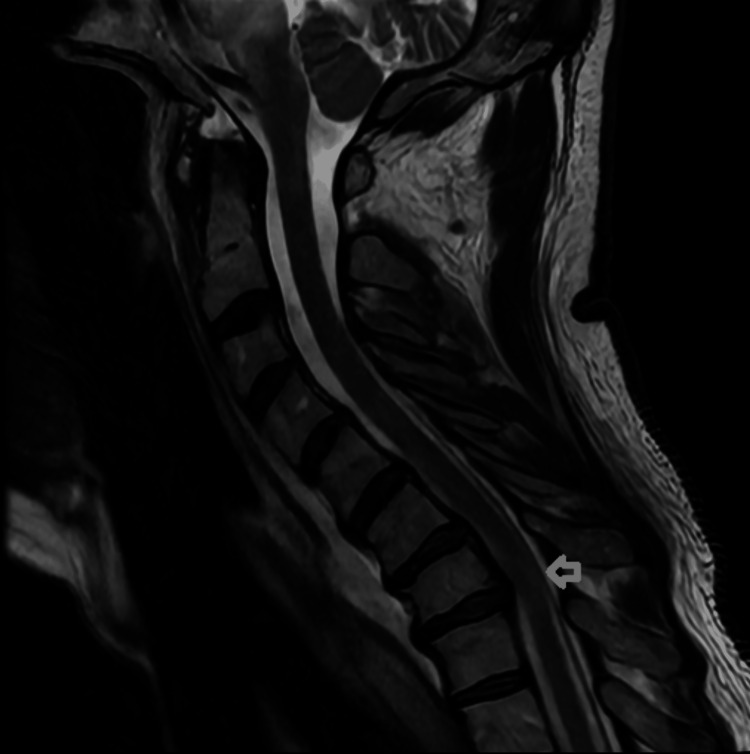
MRI C spine (T2 sequence) sagittal view demonstrating compression of the spinal cord, resulting in increased central and right lateral cord T2 signal due to suspected large right central and subarticular disc protrusion (arrow).
